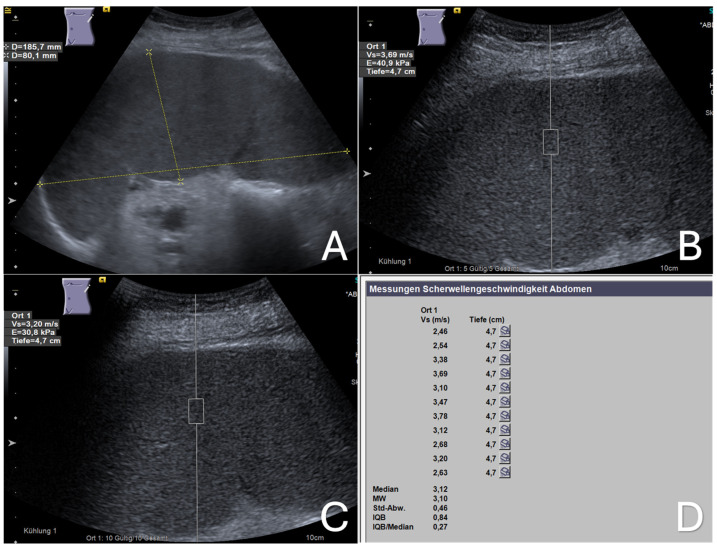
Benign congestive splenomegaly. A 52-year-old male patient with liver cirrhosis-related portal hypertension. (A) B-mode ultrasound image of the enlarged spleen with a length of 18.6 cm and a thickness of 8.0 cm; (B,C) two elastographic images of enlarged spleen showing shear wave velocities (Vs) of 3.69 and 3.20 m/s, respectively; (D) the final elastographic report of the enlarged spleen, showing a mean shear wave velocity (MW) of 3.10 m/s. Ort 1: location 1; Vs (m/s): velocity in meter per second; Tiefe (cm): depth in centimeter; MW: mean value (Mittelwert); Std-Abw.: standard deviation (Standard Abweichung); IQB: interquartile range (Interquartilbereich).
